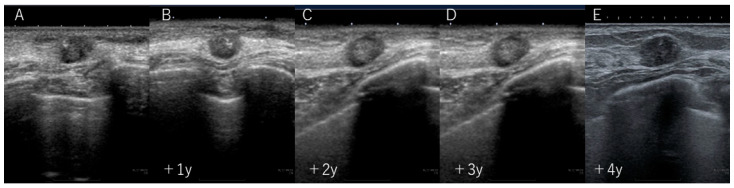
From (A) the initial ultrasound examination to (B–D) the follow-up ultrasound examinations 3 years later, no changes in the size or shape of the tumor were observed, and the diagnosis of fibroadenoma was upheld. (E) In the ultrasound examination 4 years later, the tumor size slightly increased to 11 mm.
